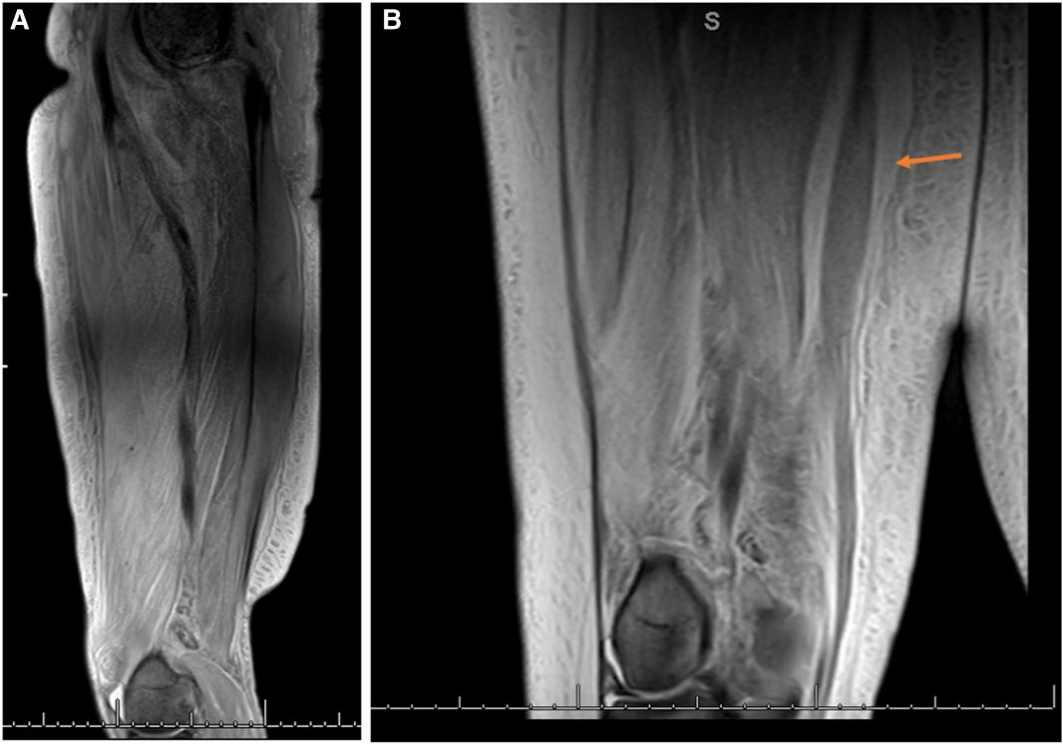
A, Selected coronal T2 magnetic resonance imaging scans showing increased signal of rectus femoris musculature. B, Fluid in the epimysium and along fascial planes (arrow) is most notable around the gracilis muscle indicative of inflammatory changes.
